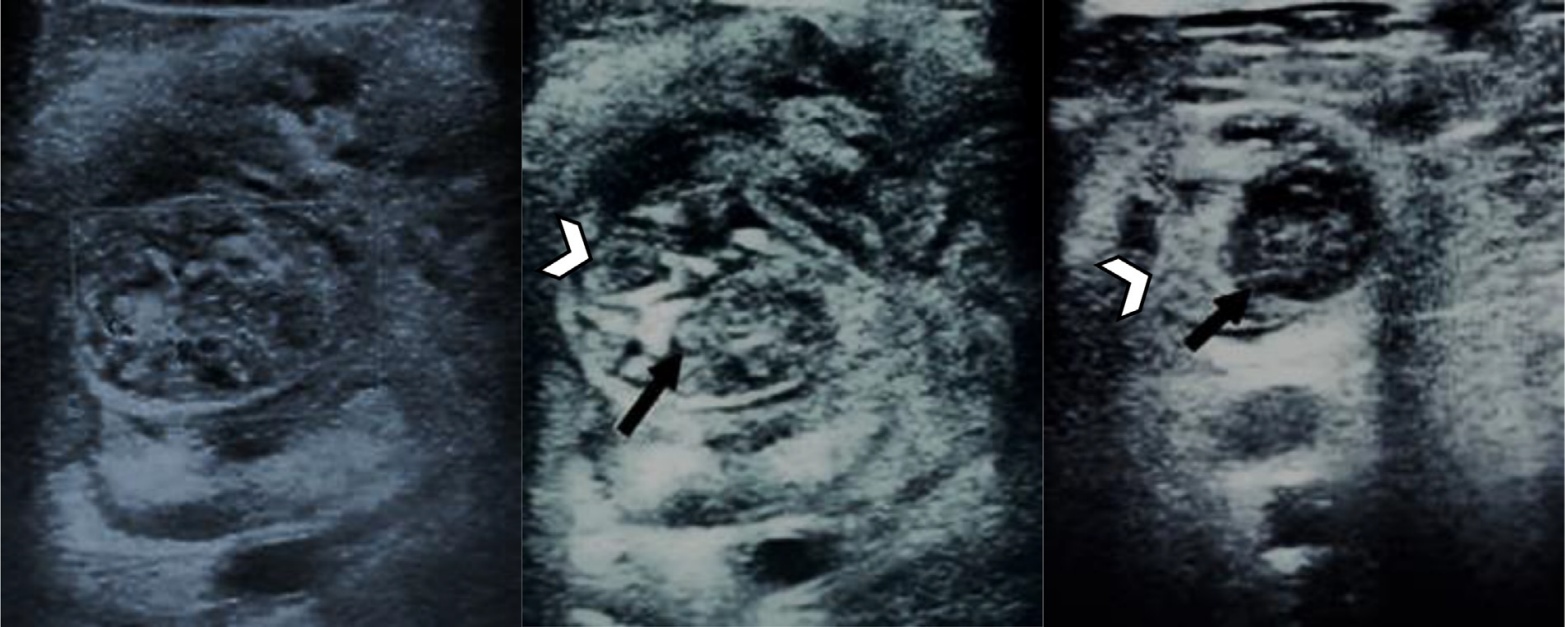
Ultrasound images of the left popliteal fossa demonstrated an enlarged and infiltrated nerve with honeycomb appearance (arrows) surrounded by a mixed echogenicity mass (arrowheads).
